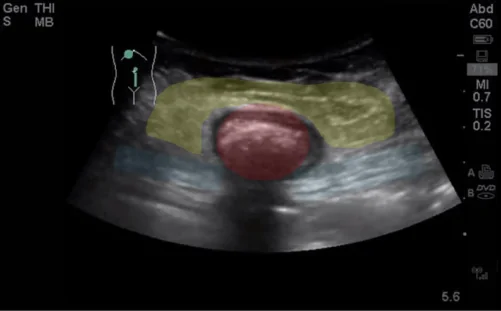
Ultrasound confirming failure of multiple attempts to manually reduce the hernia. A loop of bowel (red) is seen protruding from the abdominal muscle layer (blue) and into subcutaneous fat (yellow). A clear defect is seen in the abdominal musculature deep to the herniated bowel.
